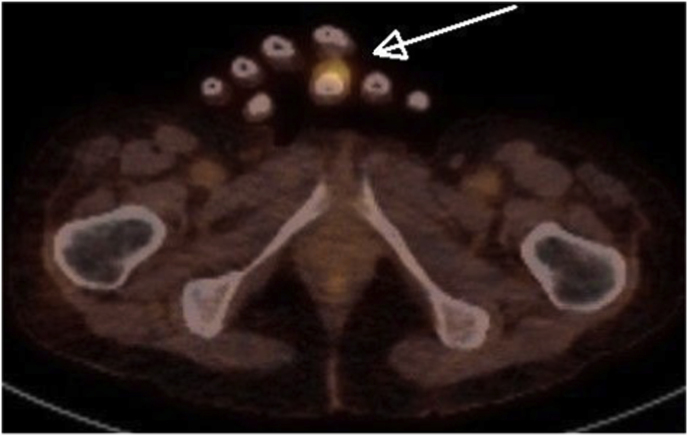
Axial plane of PET-CT with increased FDG uptake of the middle of the left hand.
